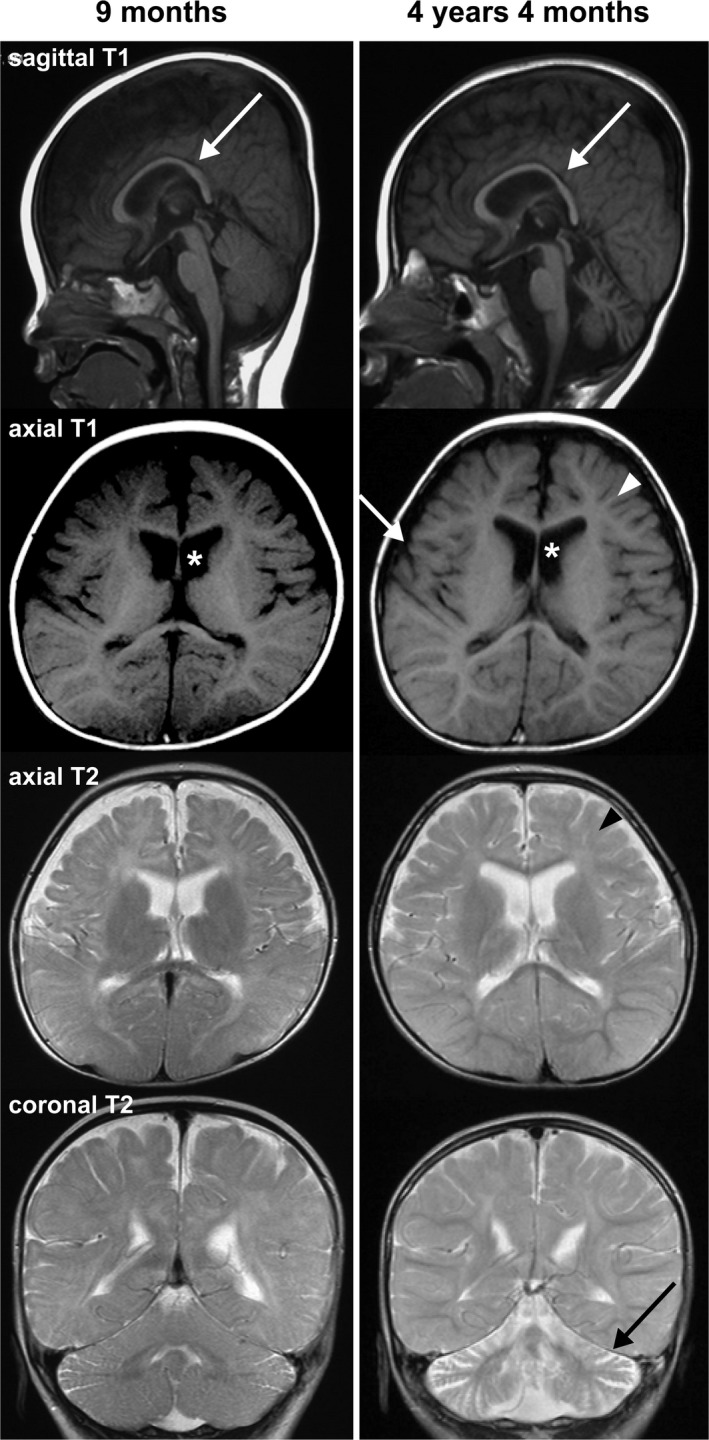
Brain magnetic resonance imaging examinations of the patient at 9 months and 4 years 4 months revealed progressive cerebral and cerebellar atrophy, and white matter abnormalities. T1‐weighted (sagittal and axial) and T2‐weighted (axial and coronal) images are shown. Malformation of the corpus callosum (sagittal T1, arrows), lateral ventricular enlargement (axial T1, asterisks), white matter abnormalities (axial T1 and 2, arrowheads), and cerebral (axial T1, arrow) and cerebellar (coronal T2, arrow) atrophy are marked in the figure
